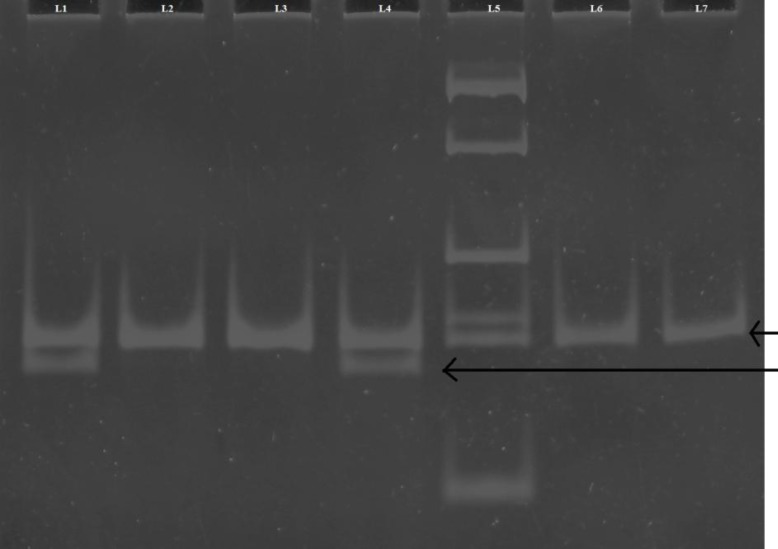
MTHFR* gene polymorphism. Polyacrylamide gel picture showing PCR-RFLP products of *MTHFR* gene. L2, 3, 6: CC (-/-) genotype; L1, 4: CT (+/-) genotype; L7: undigested PCR product; L5: 100 bp ladder
